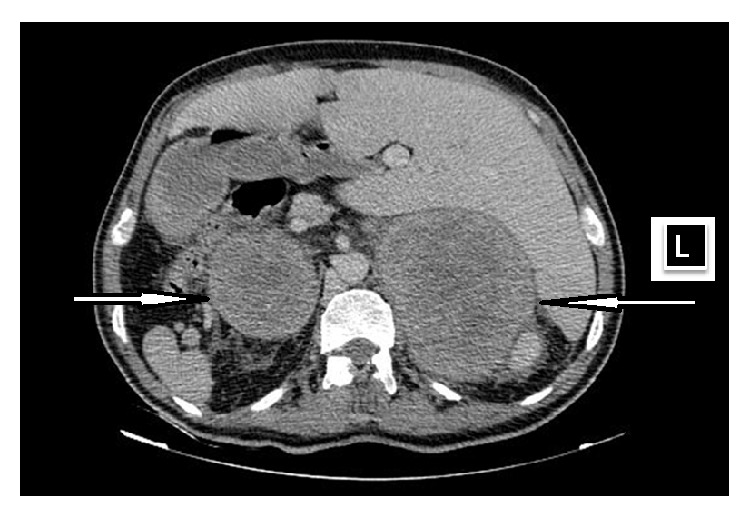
Abdomen CT image showing situs inversus and a 13 cm mass on the left adrenal gland and 7 cm mass on the right adrenal gland.
